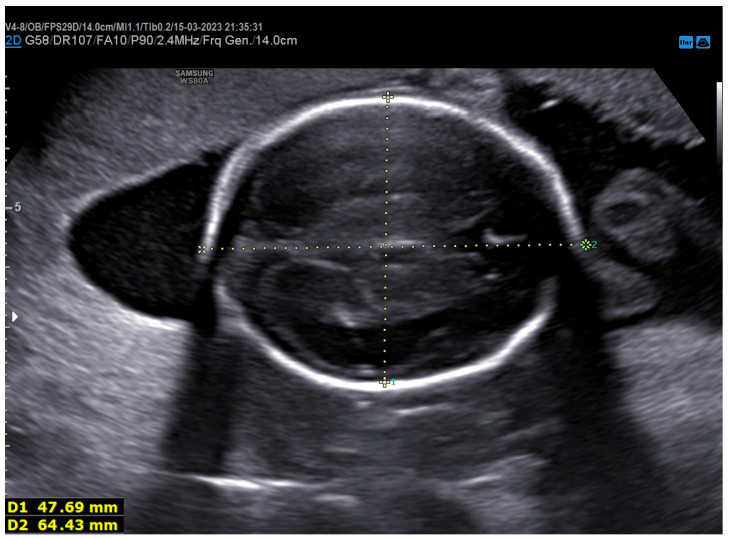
Head growth was under the 5th percentile for gestational age in the second-trimester ultrasound.
